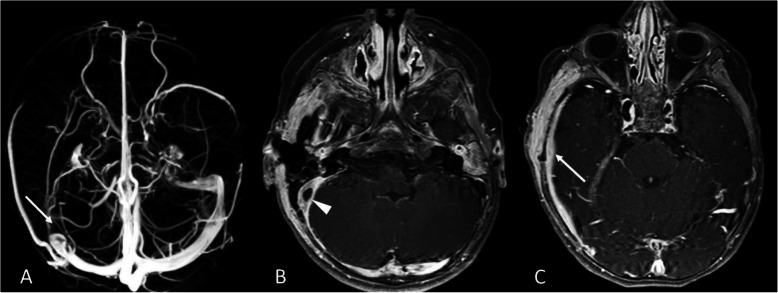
a Magnetic Resonance Venography showed the absence of the flow-related signal in the right sigmoid sinus (arrow). b The contrast-enhanced T1-weighted sequence showed the thrombosis and the wall-thickening of the right sigmoid sinus (arrowhead), as well as (c) the inflammatory thickening and the contrast-enhancement of dura mater (arrow) in the right temporal region
